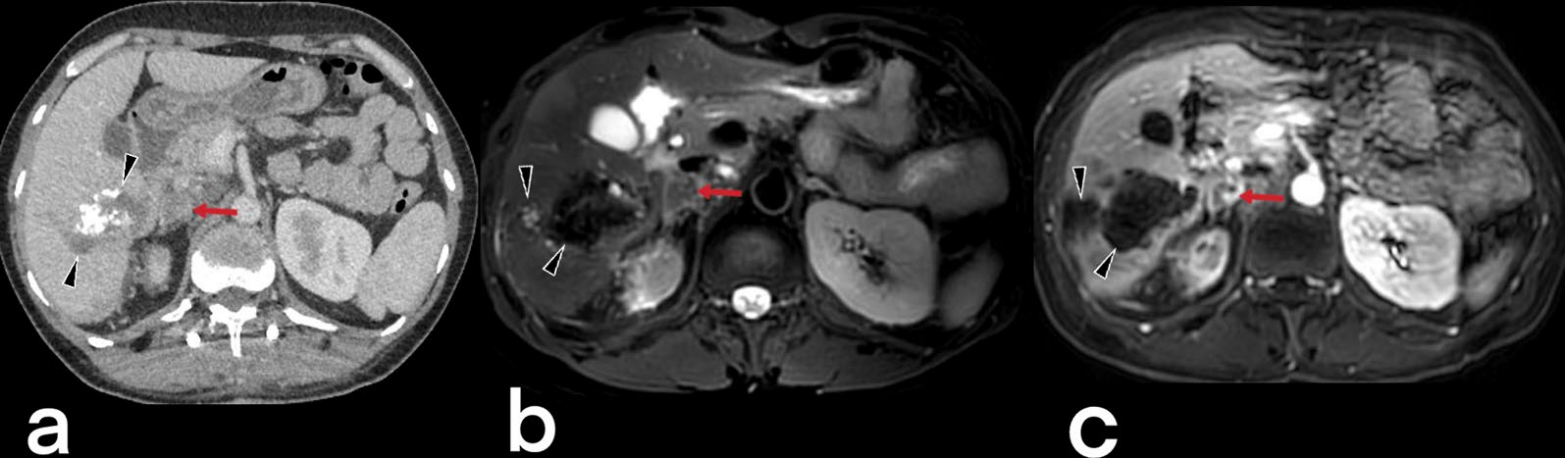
A 52-year-old female. Contrast-enhancement CT (a), T2 weighted MR (b), and contrast-enhancement T1 weighted MR (c) images demonstrate a heterogeneous lesion with peripheral enhancement (arrows) in the right adrenal gland (adrenal TB). Multiple calcified heterogeneous areas (arrowheads) are also seen in the liver (hepatic TB)
